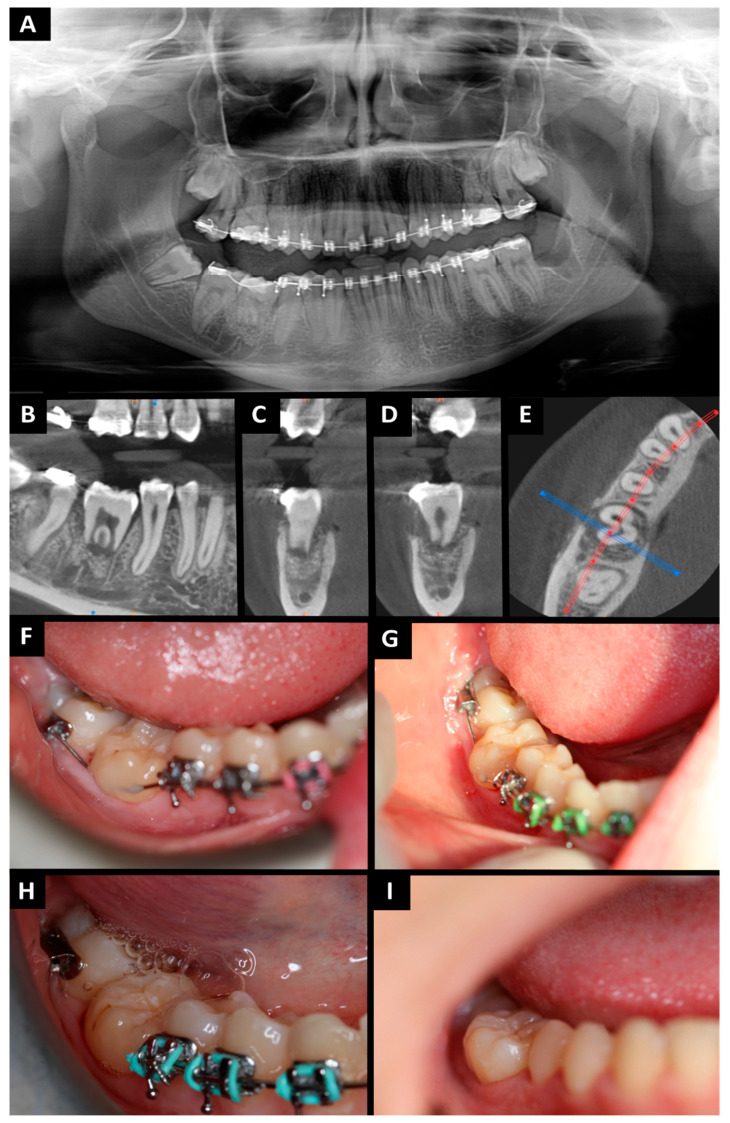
Orthopantomography (A) tooth no. 38 in place of tooth no. 46 (immediately after surgery); CBCT after 6 weeks (B–D): (B) pantomography reconstruction—tooth no. 38 in place of tooth no. 46; (C) cross-sectional view—mesial root of tooth no. 38; (D) cross-sectional view—distal root of tooth no. 38; (E) axial view—visible bone healing; (F) soft tissue healing after 6 weeks; (G) soft tissue healing after 5 months; (H) intraoral photography after 6 months; (I) intraoral photography after 6 months.
